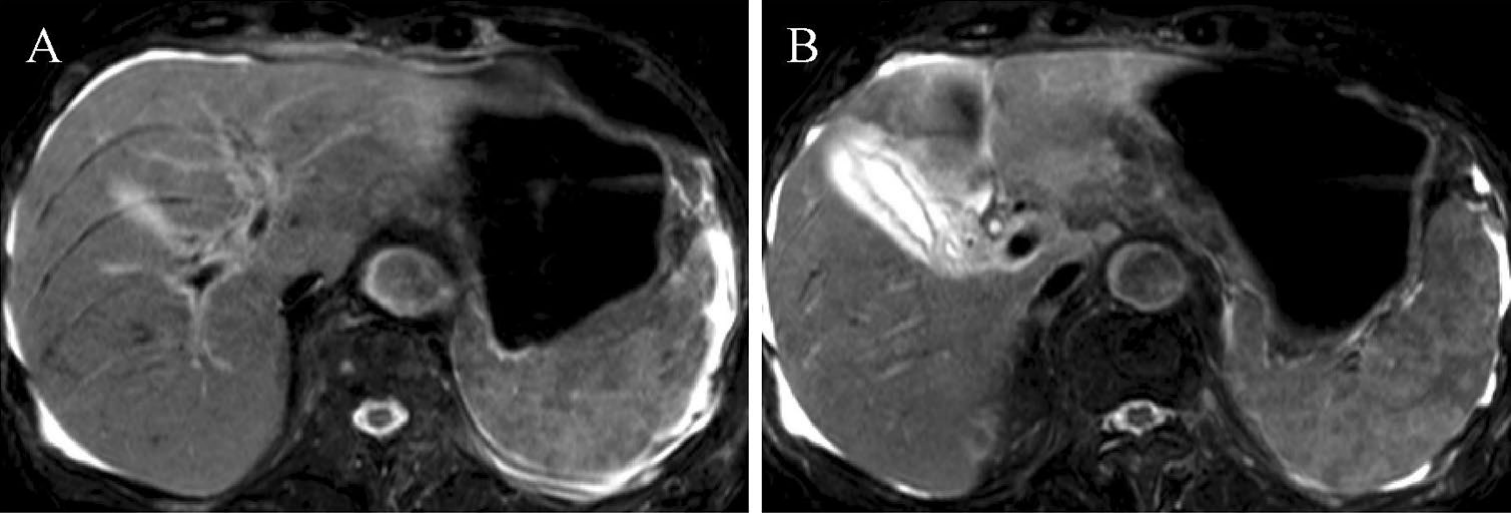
An MRI examination revealed thickening of the gallbladder wall (a) and ascites (b)
